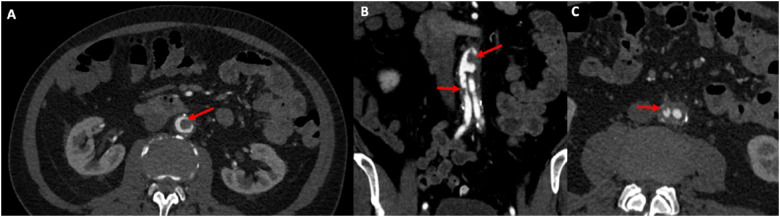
CTA post thrombolysis demonstrates a significant burden of residual thrombus within the graft (red arrows) in the aortic segment (A/B) and the right iliac limb (C).
